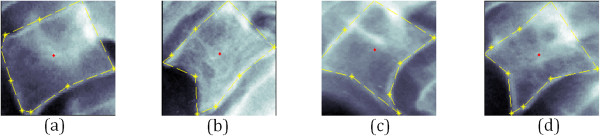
Examples of AOs classes of the cervical x-ray images. Samples of Macnab’s classification and their osteophyte severity grading in the cervical vertebrae x-ray images, (a) normal (b) moderate traction (c) severe claw and (d) slight claw-traction.
